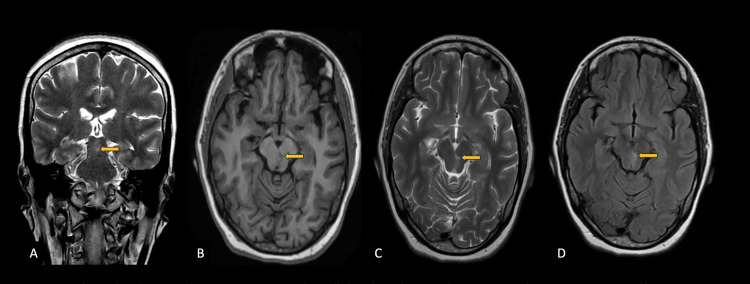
MRI Brain; T1, T2 and FLAIR axial images MRI brain; T1, T2 and fluid-attenuated inversion recovery (FLAIR) images reveal a focal altered signal intensity lesion in the left paramedian region of the midbrain (yellow arrow) appearing hypointense on T1 weighted image (WI) axial plane (B), hyperintense on T2WI in coronal (A); axial (C) and FLAIR axial plane (D).
